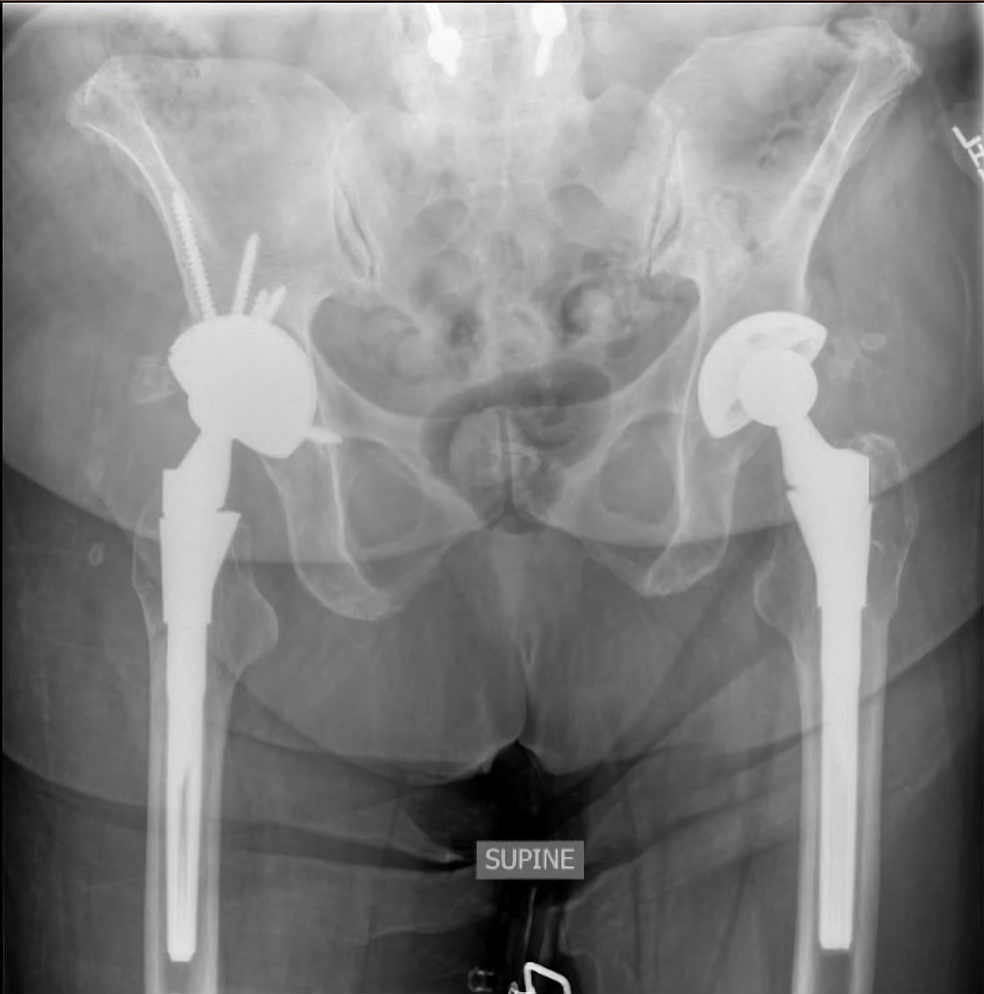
Immediate postoperative anterior-posterior pelvis radiograph This radiograph demonstrates the new S-ROM femoral prosthesis and a 58-mm multi-hole acetabular cup with 20 degrees of added anteversion. Seven screws were placed, with two of these screws being ischial S-ROM: Sivash-range of motion
